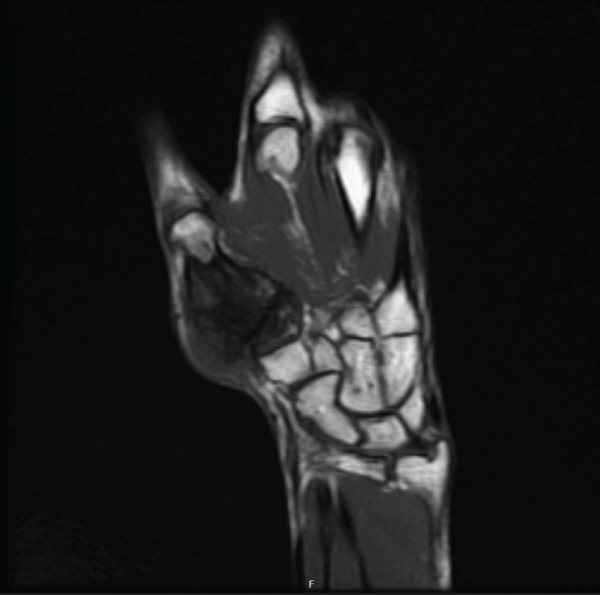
MRI of right hand showing a destructive mass involving the first metacarpal bone.
